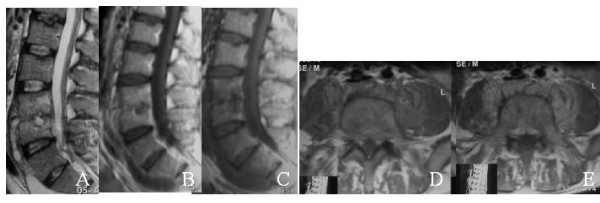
A) T2-weighted image shows increased signal intensity involving subchondral part of L4 spine adjacent to L3-4 disk and decreased signal intensity intervertebral disc space. B) T1-weighted image shows iso-to hypointence signal intensity. C) T1-weighted image after contrast injection shows decreased signal intensity intervertebral disc space. D) T1-weighted axial image shows paravertebral irregulary mass with iso-to hyperintence signal intensity. E) T1-weighted axial image after contrast injection shows increased signal intensity paravertebral mass.
